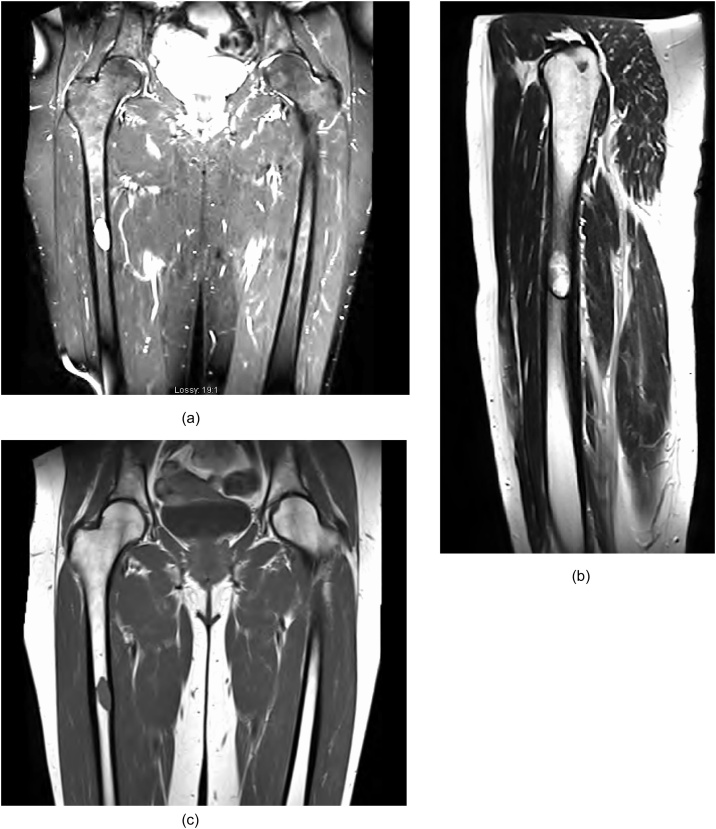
MRI with standard multi-planar technique*. (a, b) Coronal and sagittal T2 weighted MRI images of a 1.4 × 2.0 × 3.3 cm showed a hyperintense, well marginated, eccentrically located and marginally expansile lesion involving the mid right femoral shaft. (c) Coronal T1-weighted image showed a well circumscribed cystic lesion of the right femoral shaft with a narrow zone of transition. It was fluid filled and has a relatively homogenous consistency with no cortical expansion. No other focal lesion seen.
